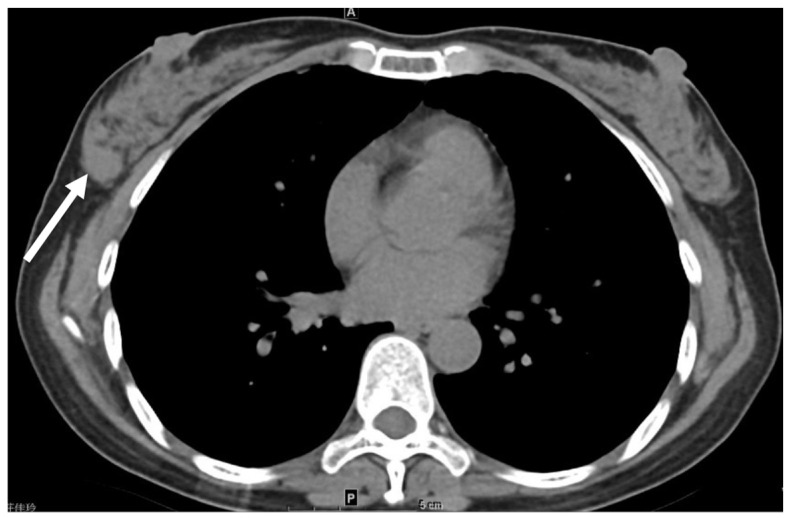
An incidental solid breast nodule detected in a low-dose computed tomograph (LDCT). An axial LDCT of a 48-year-old female shows a 1.5 cm solid nodule in the right outer breast (arrow). The nodule was proven to be breast cancer after surgery and pathology. (A, anterior. P, posterior).
